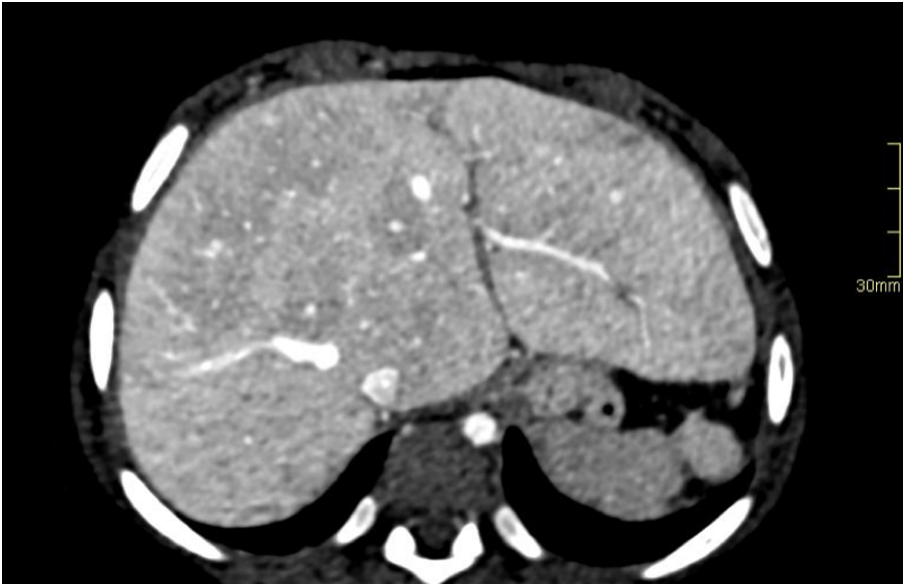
Hypoplastic left heart syndrome with Fontan circulation in a 4-year-old girl with failing Fontan. Abdominal CT with i.v. contrast, portal venous phase, in an axial plane reveals hepatomegaly with round hepatic borders and perisinusoidal edema. A biopsy performed pretransplantation, revealed fibrosis grade 3–4. Note the small spleen due to previous infarction.
